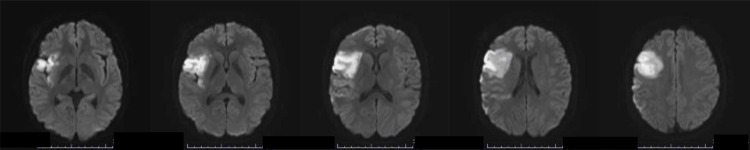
Brain MRI performed on day 51. Axial diffusion magnetic resonance imaging showed new high-intensity signals in the right frontal lobe.
